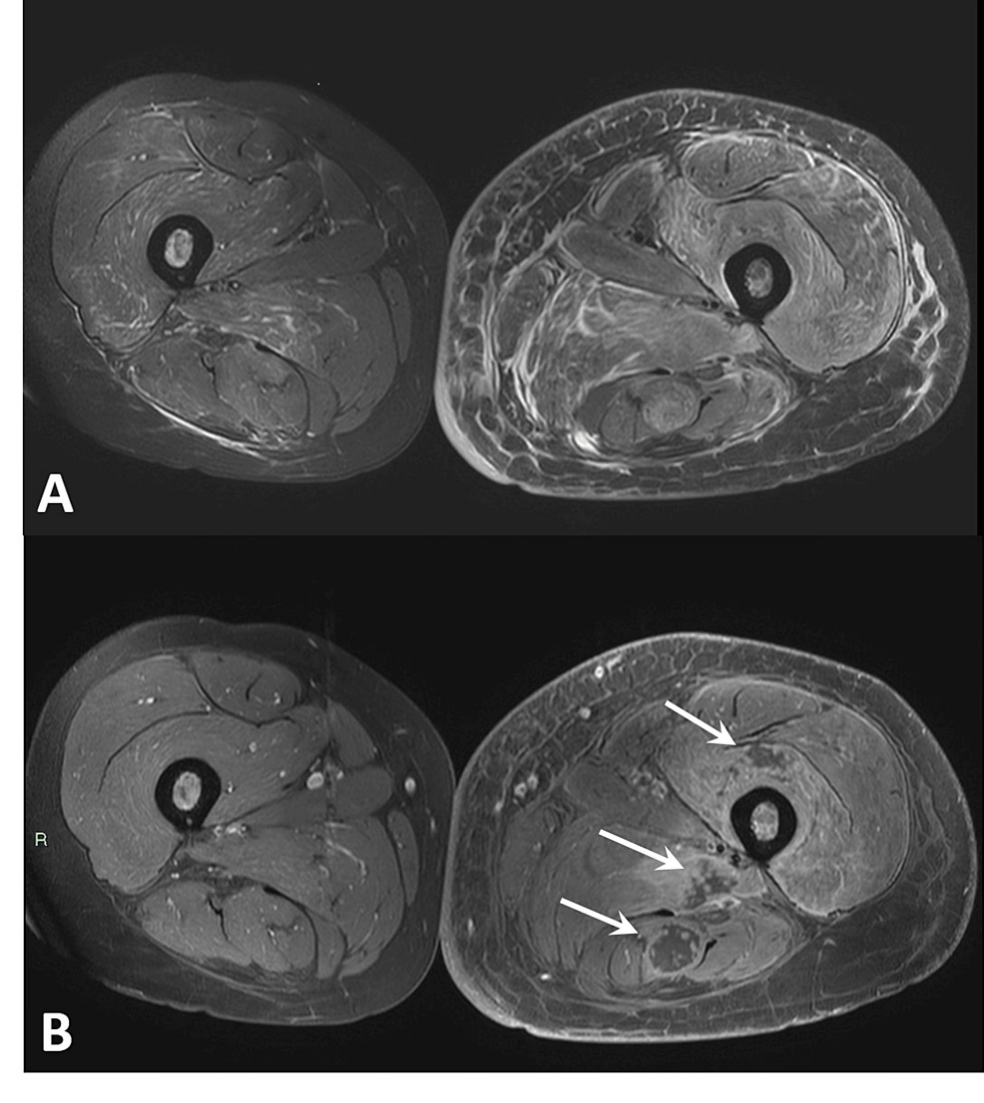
Magnetic resonance imaging of the thigh. (A) Axial T2-W with fat suppression and (B) T1-W with fat suppression after intravenous (IV) gadolinium injection MRI images of the thighs. Massive muscle edema involving the majority of the muscles of the left thigh is observed. Following contrast injection, multiple round myonecrotic lesions (arrows) are seen along the tract of the radiation beam.
